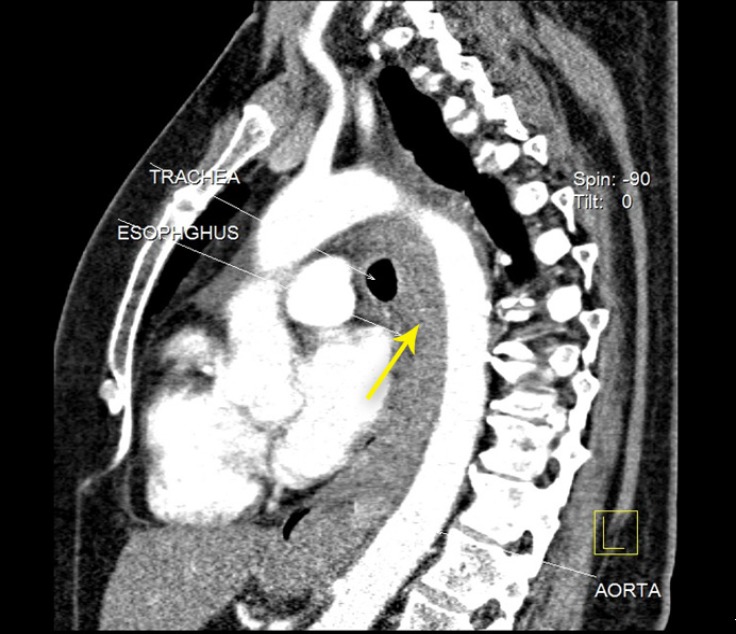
Computed tomography angiography, showing a large mass-like area along the posterior aspect of the thoracic esophagus (arrow)
